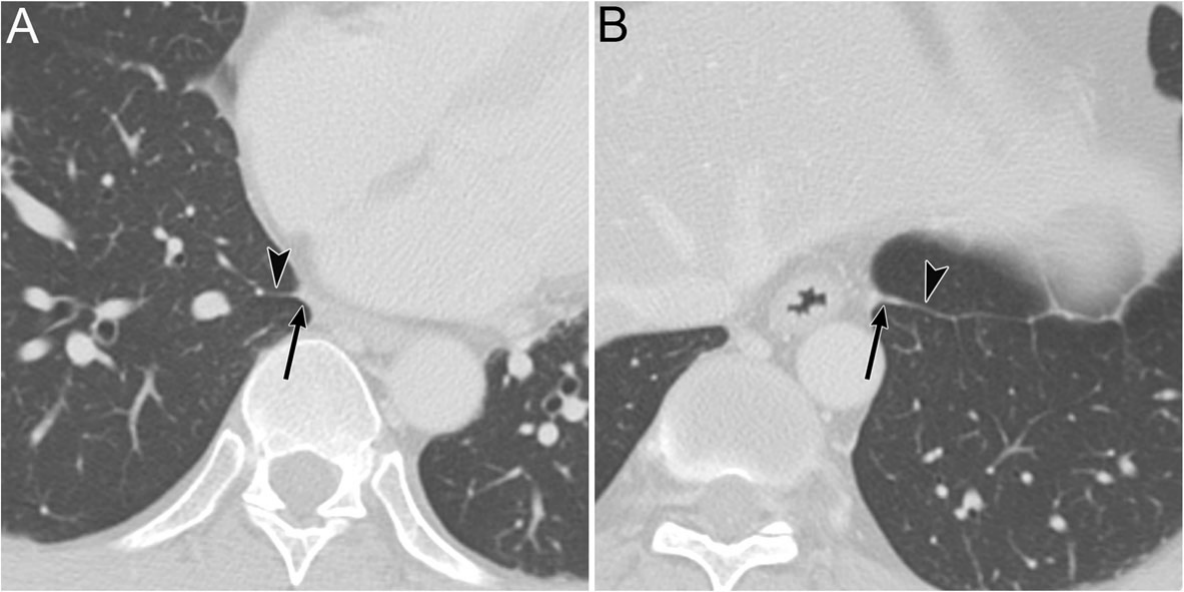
The normal inferior pulmonary ligament (IPL) on CT. A 48-year-old man with chronic obstructive pulmonary disease. a An axial CT image of the right lung at the lung base shows the right IPL (black arrow). It appears as a beak that extends laterally from the mediastinum (between the IVC and the azygos vein) with the intersublobar septum (black arrowhead). b An axial CT image of the left lung at the lung base shows the left IPL (black arrow). It appears as a beak that extends laterally from the mediastinum (along the esophagus) with the intersublobar septum (black arrowhead)
